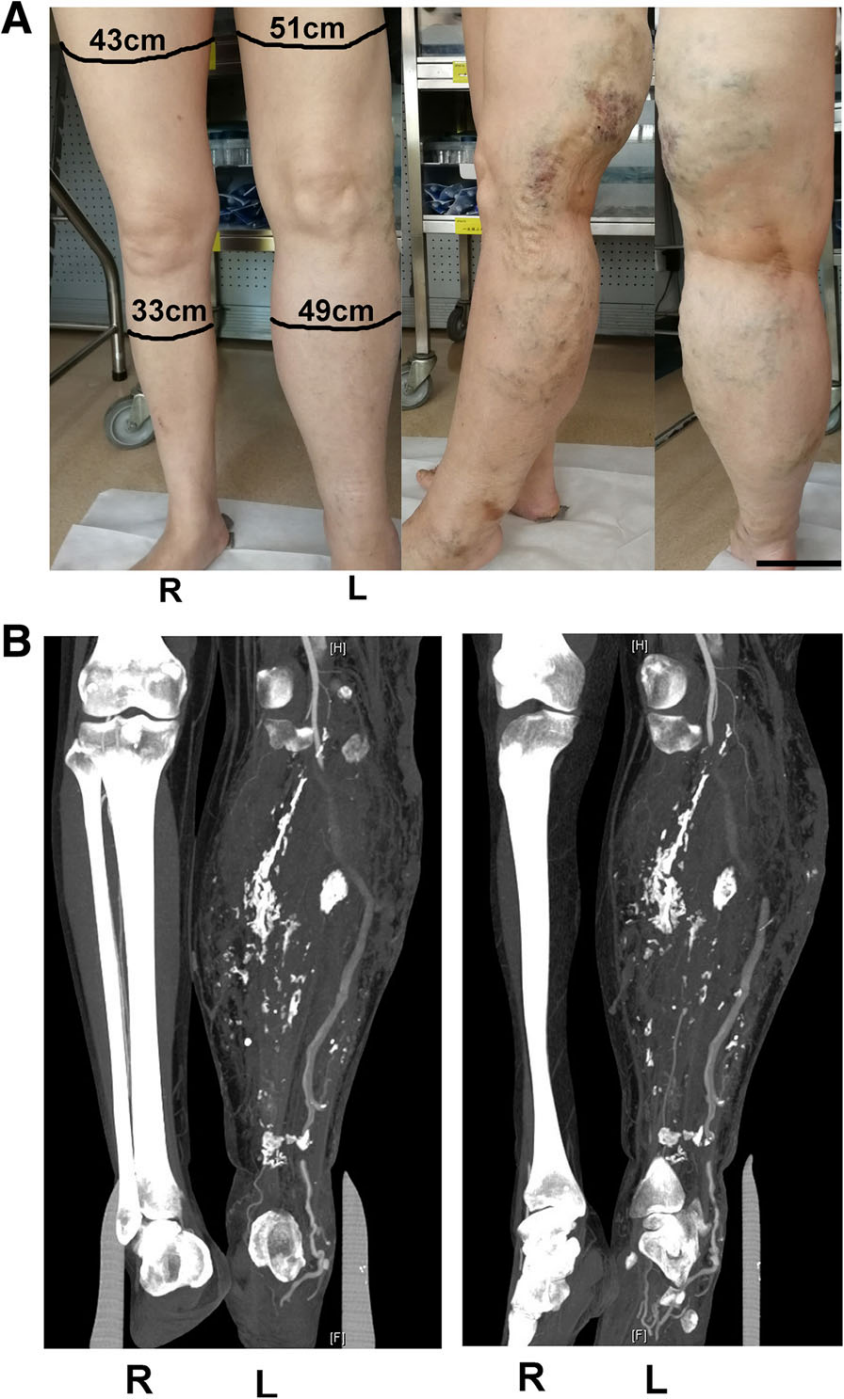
Lesions in the left lower limb. a General morphology of the legs of the patient. Front position: the right leg was larger than the left leg, and the circumference is shown in the figure. Lateral position: numerous varicosities and rough skin on the left leg. b Contrast-enhanced computed tomography (CT) scan of the lower limbs: front and lateral positions. R: right leg; L: left leg
